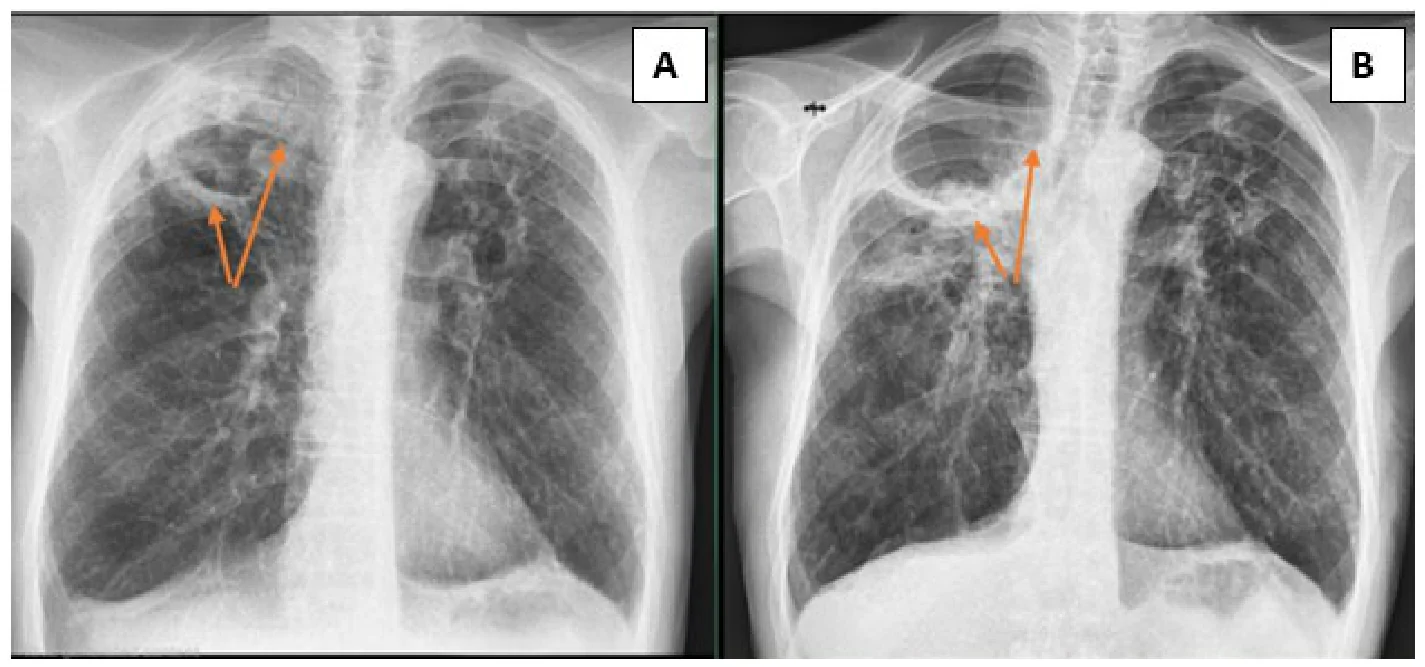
Posteroanterior chest radiographs. (A) Initial evaluation, showing the right apical cavitary lesion with intracavitary opacity/content (orange arrows). (B) Follow-up radiograph performed four months after treatment initiation, showing a slight reduction in the intracavitary content of the right apical lesion (orange arrows), while the overall radiographic appearance remains largely comparable to the previous examination.
